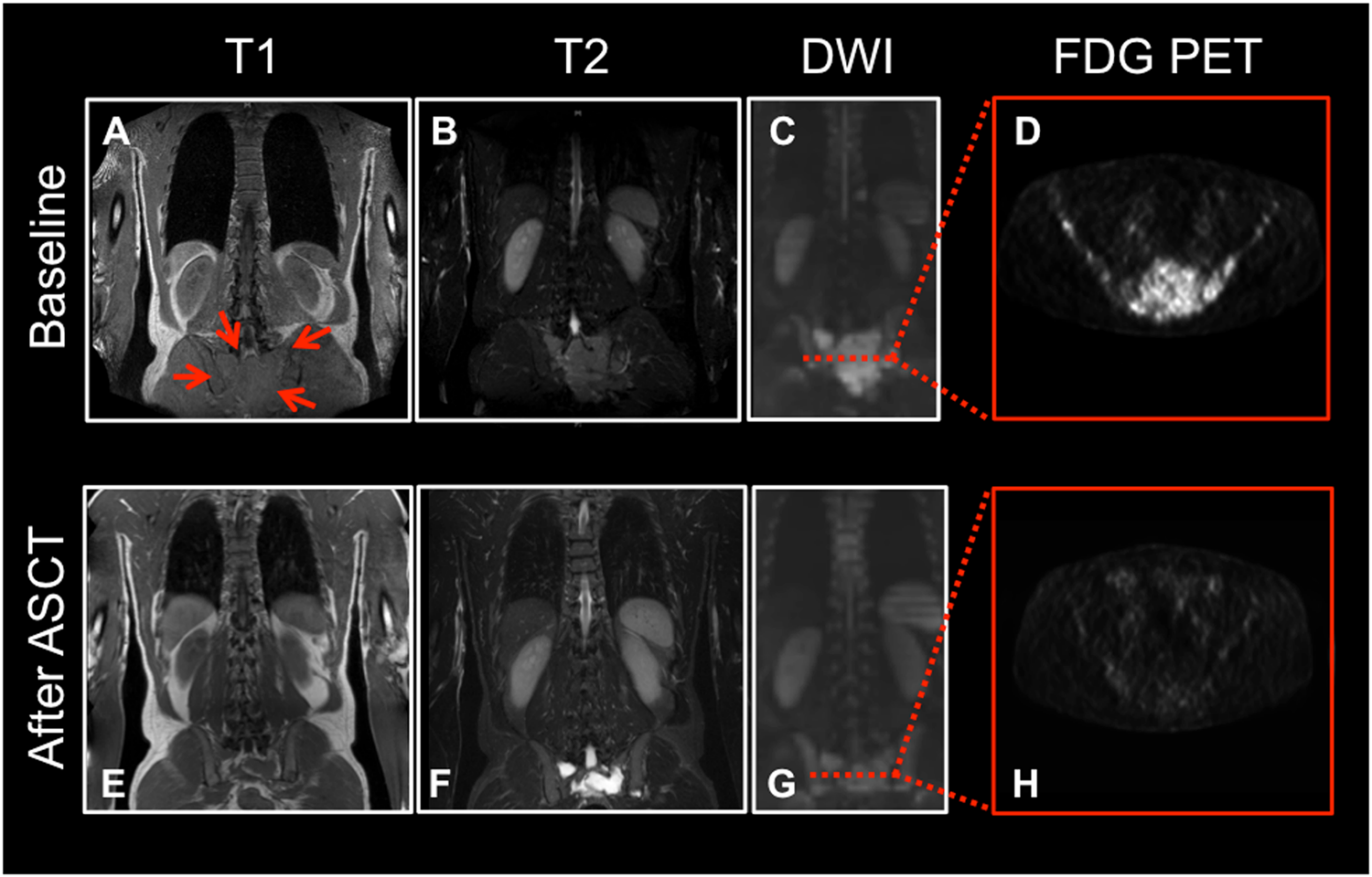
Cystic transformation of FL. Representative images from a patient with a large focal lesion in the sacrum with extramedullary growth. Images at baseline in the top row with common features of a focal myeloma lesion (red arrows): Decreased signal intensity on T1-weighted images (a) and increased intensity on T2-weighted images (b) as well as DWI (c). PET/CT proved increased FDG uptake at primary diagnosis. Upon achieving remission after ASCT (bottom row), the lesion decreased significantly in size as depicted on T1- (e) and T2-weighted (f) images. The lesion showed a hyperintense signal on T2-weighted images but no increased FDG uptake compared to the surrounding tissue. MRI images in coronar orientation, PET/CT in transversal orientation
